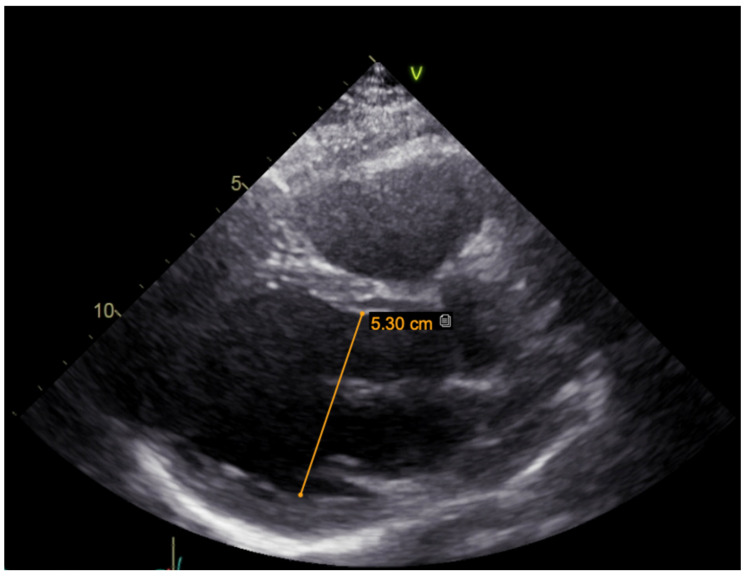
TTE parasternal long-axis view after combination treatment (improvement in LV function, LVdd 53 mm).
